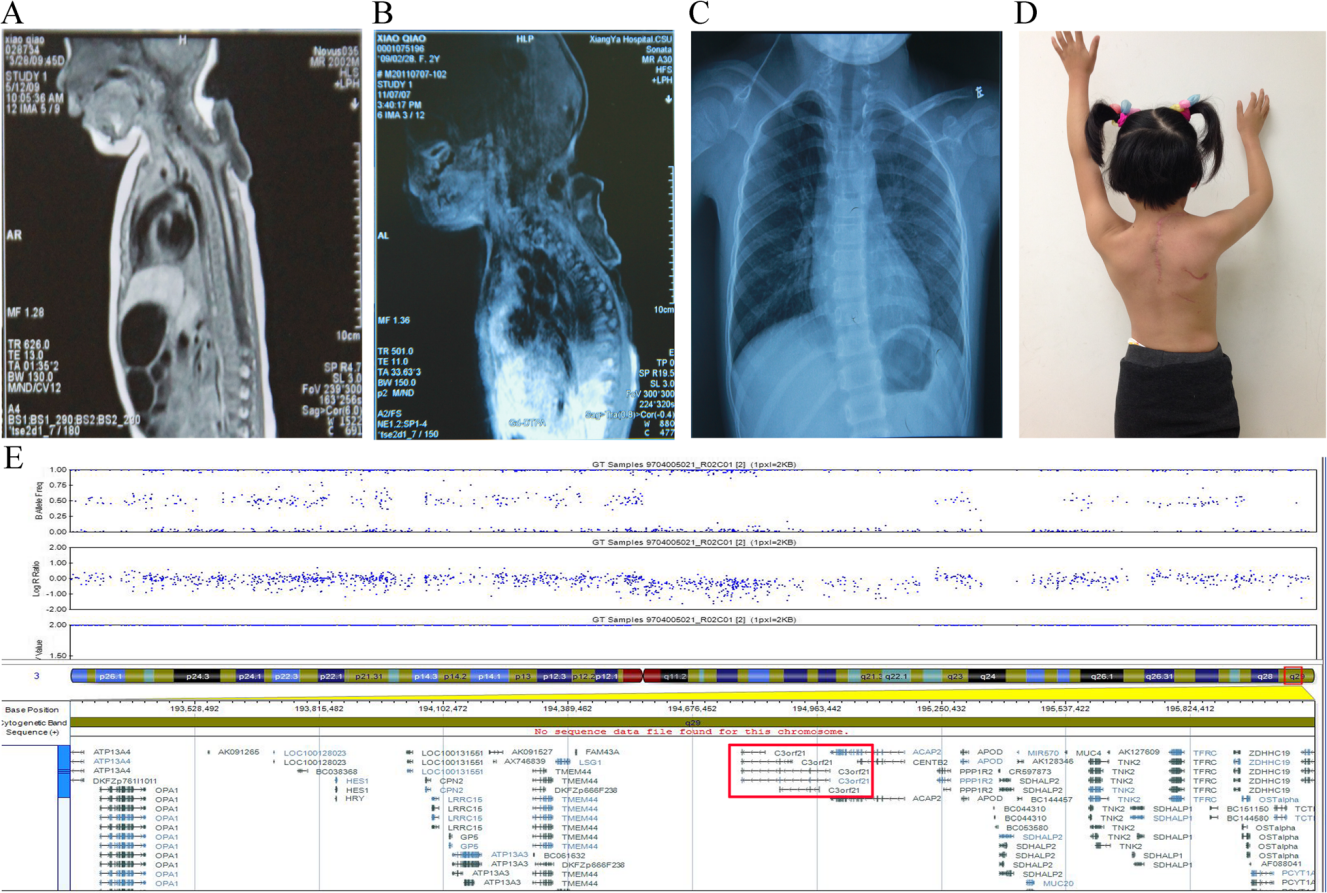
Phenotypic appearance of the affected individual in our study. a X-ray shows spinal meningocele in the cervicothoracic region in our patient at birth. b This X-ray was taken in 2011 when the proband came to our institution for spina bifida repair. It shows that besides myelomeningocele, the proband also had elongated and descended cerebellum. These results led to her diagnosis of Chiari malformation. c Patient’s X-ray from 2016, revealing an elevated right scapula. d After partial scapulectomy and collum costatectomy, the shoulder function was partially restored. e Human 660w–Quad SNP-array results. A 666 Kb microdeletion (chr3:194,532,035–195,198,585; Hg19) located in the 3q29 region. *XXYLT1* (*C3orf21*) is affected by this deletion
